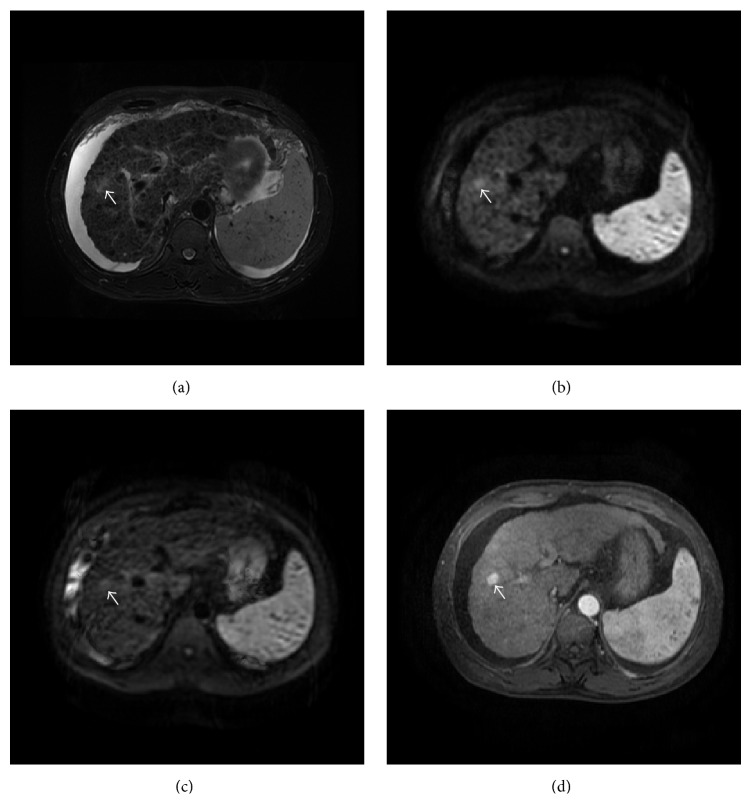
Transverse images in 62-year-old man with HCC. (a) Fat-suppressed T2-weighted fast spin-echo image shows that lesion is difficult to be detected. (b) DW image with higher *b*-value shows that lesion is strongly hyperintense to the liver. (c) DW image with low *b*-value shows that lesion is obscure. (d) Contrast-enhanced T1-weighted image in arterial phase demonstrates strong enhancement of lesion.
